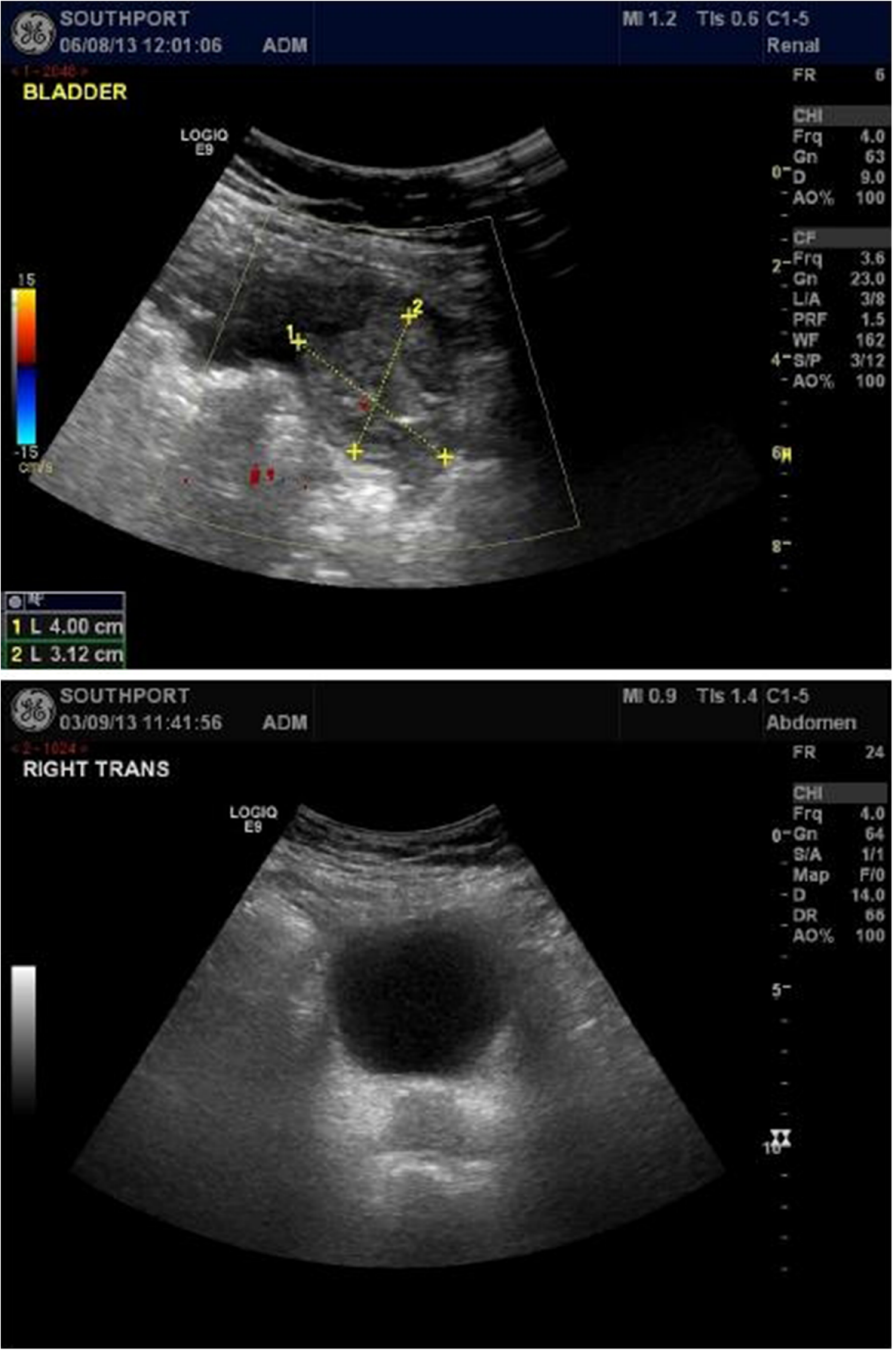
Top panel: Ultrasound scan of urinary bladder of patient # 3 was reported to show a large 4 cm × 3 cm soft tissue mass in the urinary bladder. Bottom panel: Follow-up ultrasound examination of urinary bladder performed by a senior Radiologist: Suprapubic catheter was in right position. Outline of urinary bladder was normal; there was no neoplastic filling defect or calculus.
